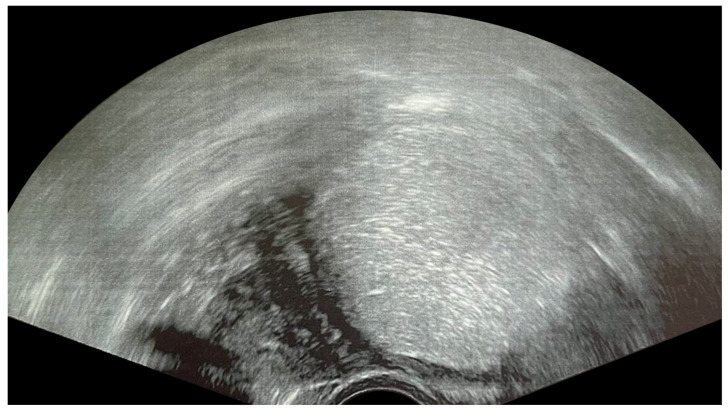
Ultrasonogram of a 17-year-old patient showing a typical unilocular endometrioma with ground-glass echogenicity of the cyst fluid.
